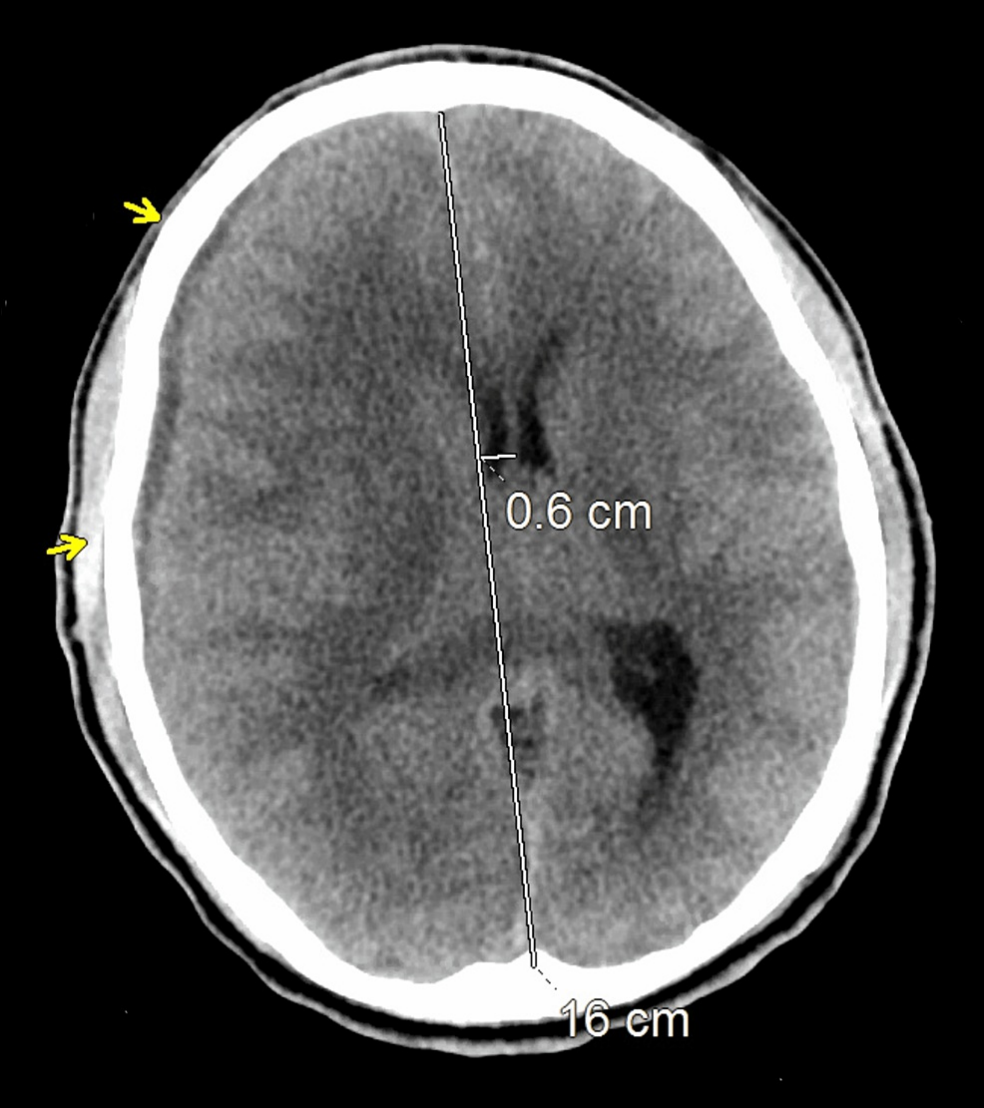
Head CT scan upon presentation to the Emergency Center: Relatively small volume hypodense subdural fluid collection on the right, mass effect with right-to-left shift of midline measured at 6mm with some mild collapse of the right lateral ventricle. CT: computed tomography
